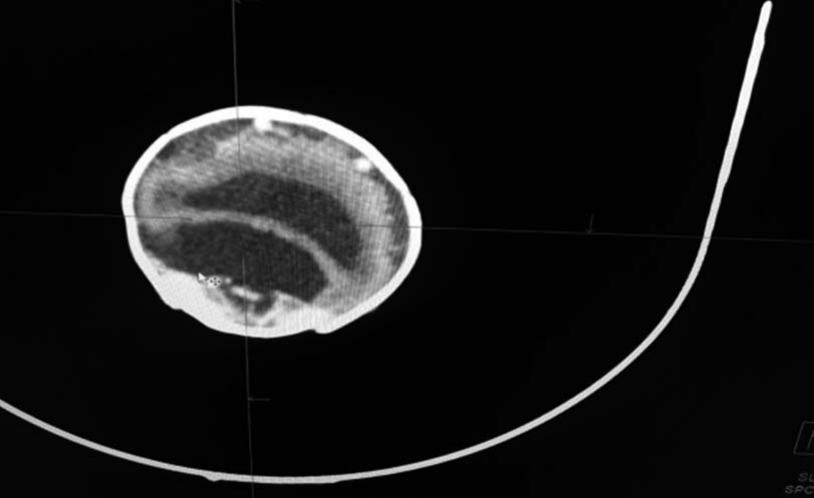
Computed tomography scan shows a single, severely dilated ventricle in the midline of the brain. Cisterna magna is very dilated. There are no clear anatomical landmarks of corpus callosum, thalamus or falx cerebri.
